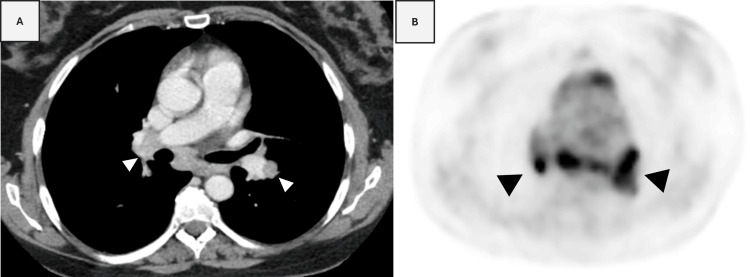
A) CT imaging also showed mediastinal and hilar (arrowhead) enlarged lymph nodes. B). PET scan images confirmed the presence of hypermetabolic mediastinal and hilar lymphadenopathies (arrowhead), which were interpreted as evidence of metastatic disease.
